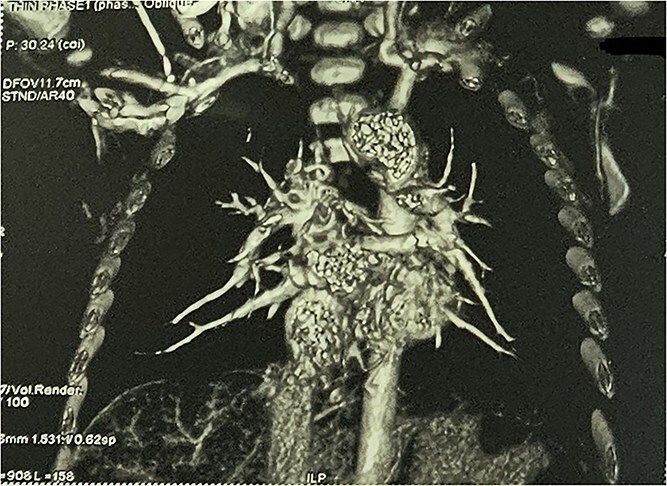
CTA demonstrating reduced distal pulmonary arborization.
